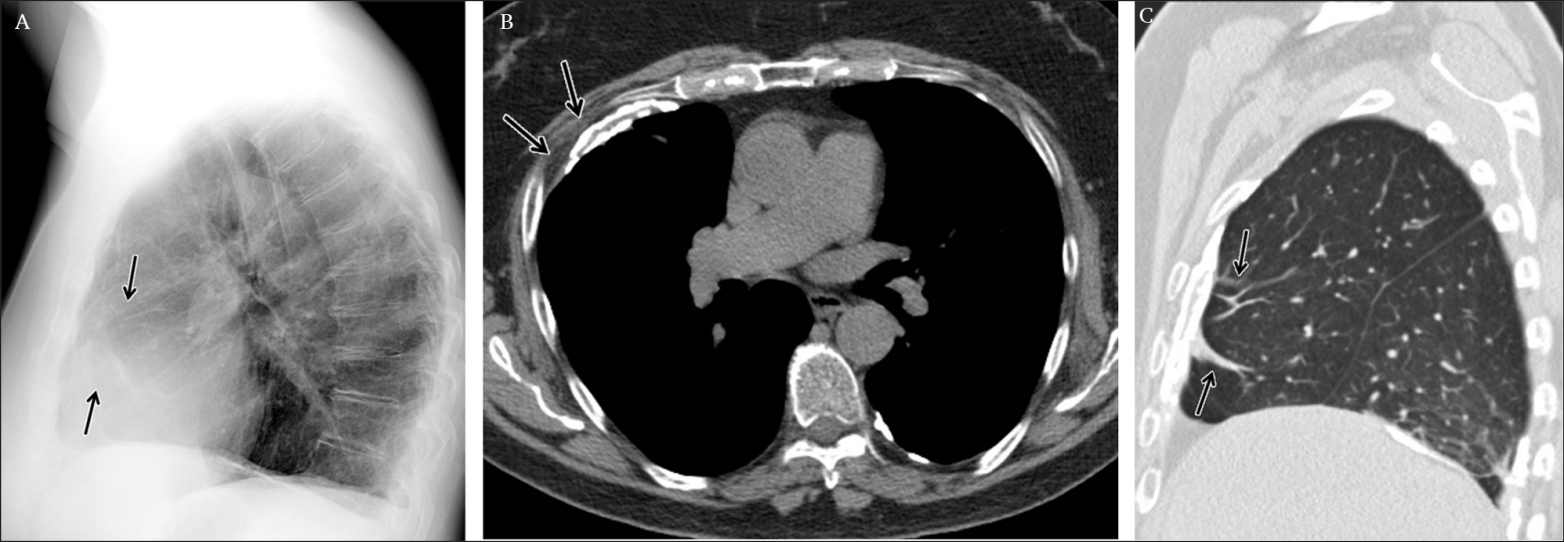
Diffuse Pleural Thickening in a 63-year old man with a history of asbestos exposure – Lateral chest radiography (a) shows a smooth uninterrupted pleural opacity extending over at least a fourth of the anterior chest wall. Note some small linear opacities in the adjacent lung parenchym(arrows). Axial CT image in soft tissue window (b) demonstrates a partially calcified pleural thickening associated with a hypertrophy of the subpleural fat (arrows). Sagittal CT image in lung window (c) shows parenchymal bands adjacent to the pleural thickening as a result from the fusion of the two pleural layers.
